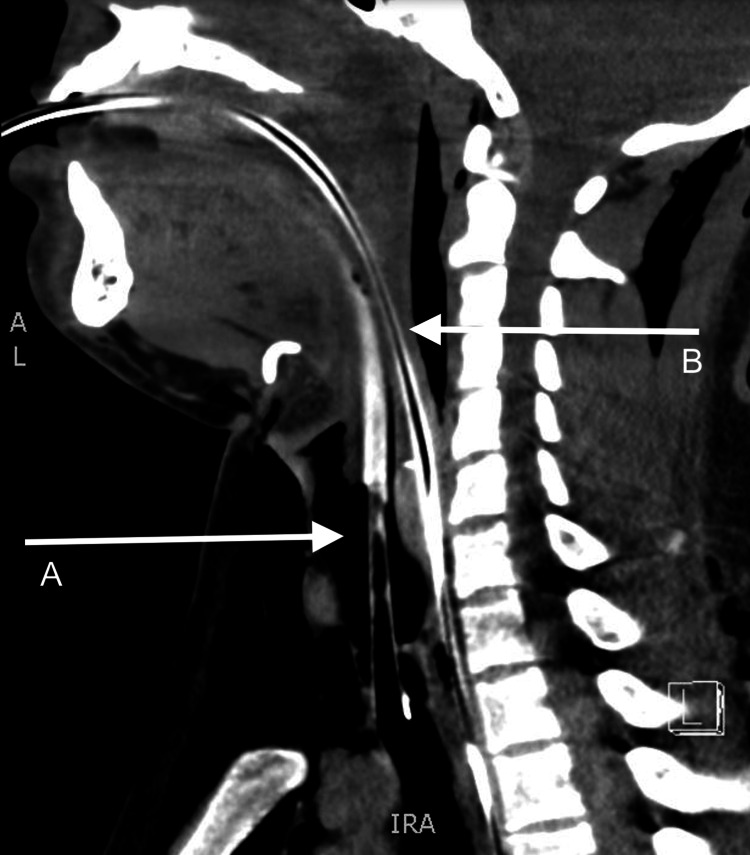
CT of the neck with arrow A pointing to an intact esophagus and arrow B pointing to ETT in the intact trachea ETT: endotracheal tube
